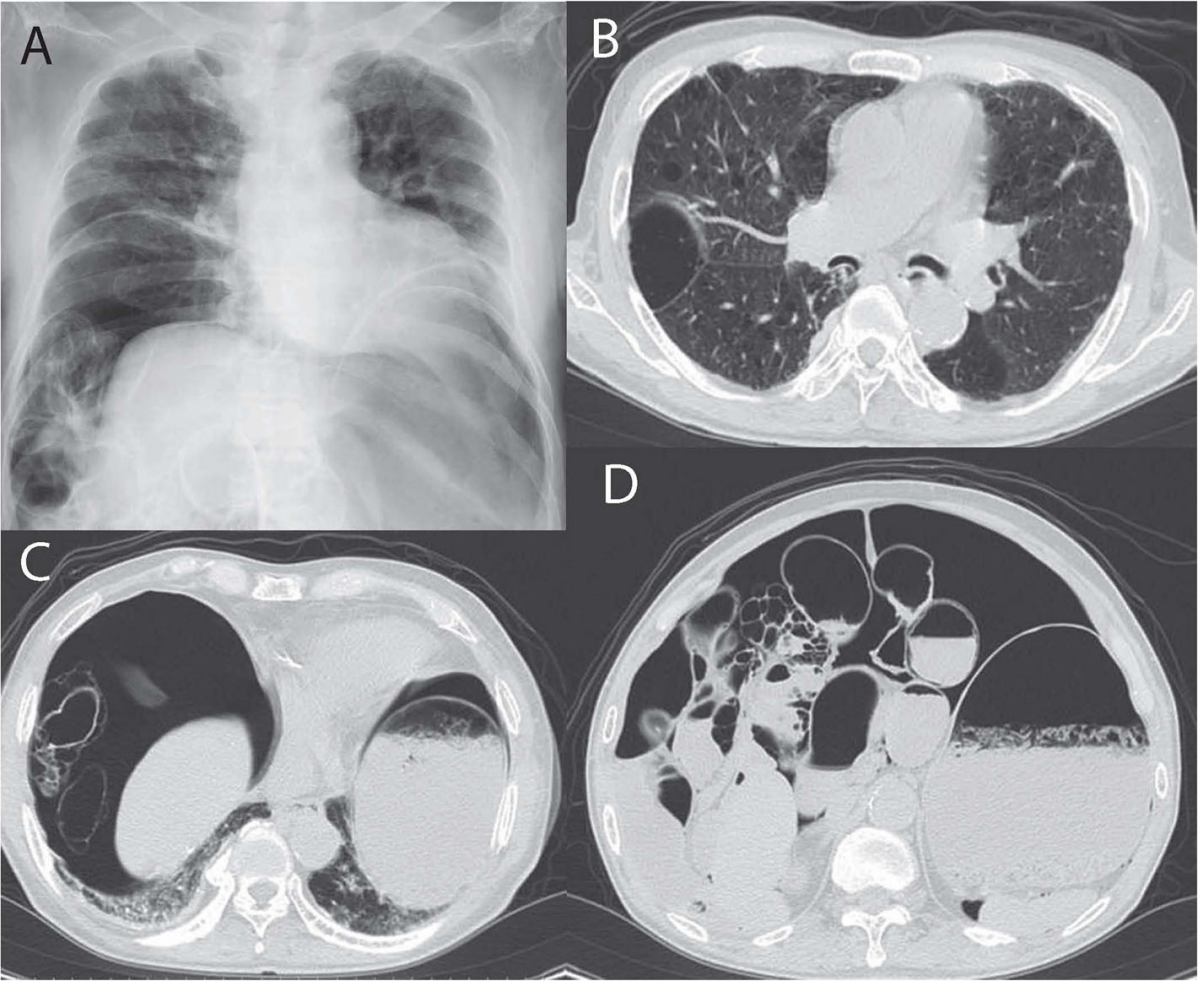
a Plain chest radiograph taken on admission indicated pneumoperitoneum. b Chest computed tomography (CT) revealed pulmonary emphysema with multiple cystic changes. c, d Abdominal and pelvic CT demonstrated pneumatosis cystoides intestinalis in the ileum and distal ascending colon associated with pneumoperitoneum
